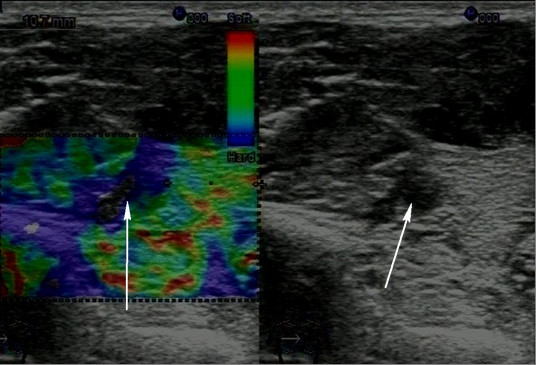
Ultrasound visualization of the affected muscle in patient with hereditary myopathy. The case shows contracted muscle tissue (hypoechoic) vs. background of increased tissue echogenicity (bright) (arrows).
